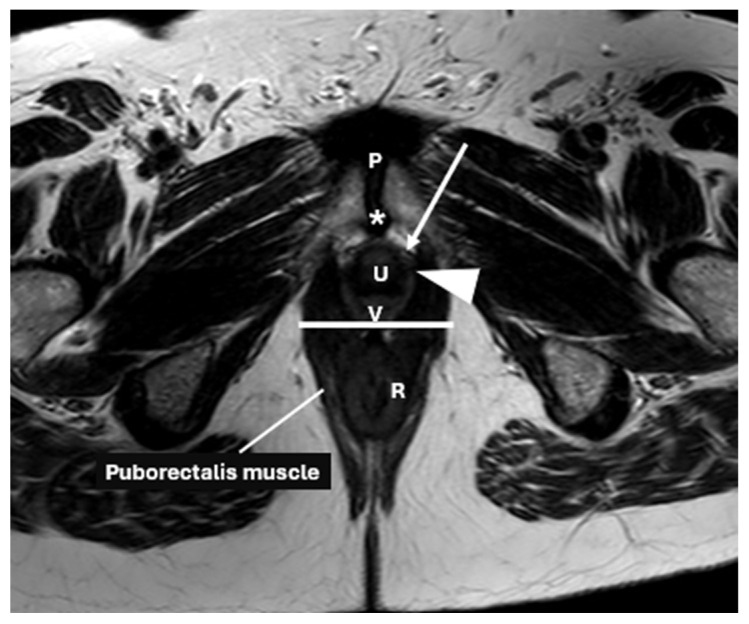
Normal female pelvic floor anatomy on axial MRI. Axial T2-weighted image shows the urogenital hiatus (white line) and key urethral support structures, including periurethral (arrow), paraurethral (arrowhead), and pubourethral (star) ligaments, with characteristic H-shaped vagina (V), urethra (U), pubic symphysis (P), and rectum (R).
